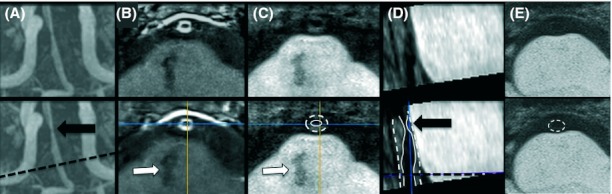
HRMRI of basilar atherosclerosis (top row unmarked and bottom row marked). (A) MRA of stenosis (black arrow) and nonstenotic artery at level of the pontine infarct (black dashed line); (B) enhancement of artery at level of infarct (white arrow); (C) FLAIR sequence with arterial wall thickening at level of the infarct on axial; and (D) sagittal views (outer wall [dashed white line] and lumen [thin white lines]) with focal stenosis above the level of infarct; (E) FLAIR images from a normal basilar artery with barely visible wall.
